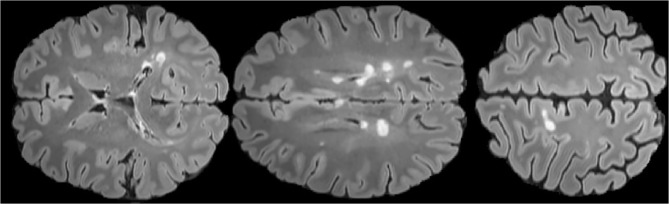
FLAIR axial MRIs of brain slices with MS lesions (white area) The figure comes from the public data set MICCAI2016 (Commowick et al., 2018).
